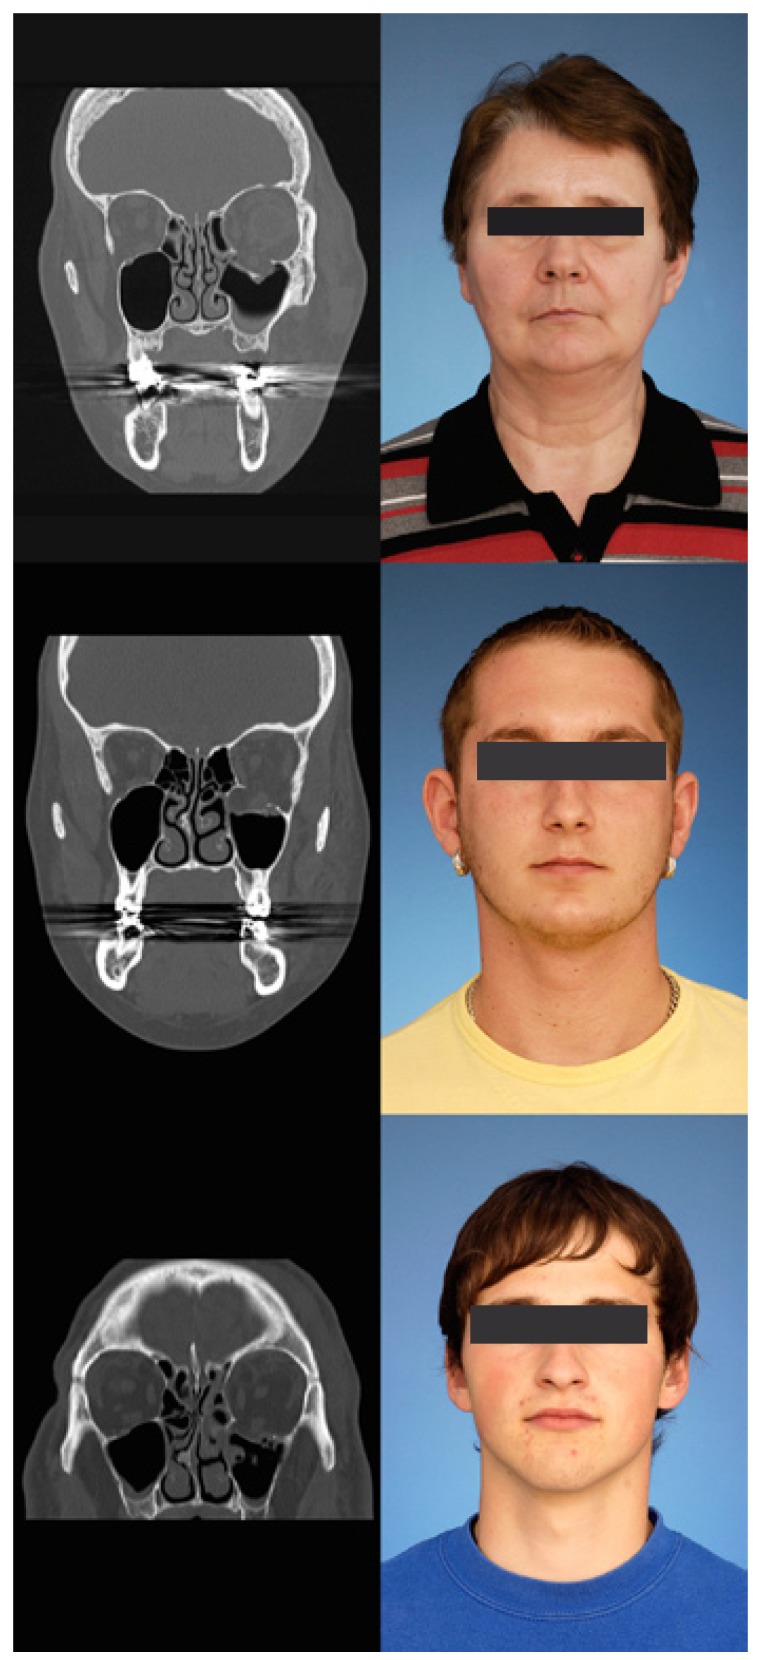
On the left coronar CT scan, on the right standardized photography three months after surgery. The patients above and in the middle were operated through a transconjunctival approach, the patient below through a subciliary approach.
